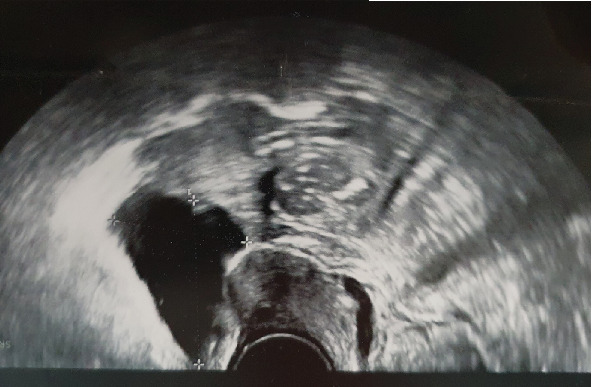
Transvaginal sonography findings in the left adnexal region after the second PID episode.
